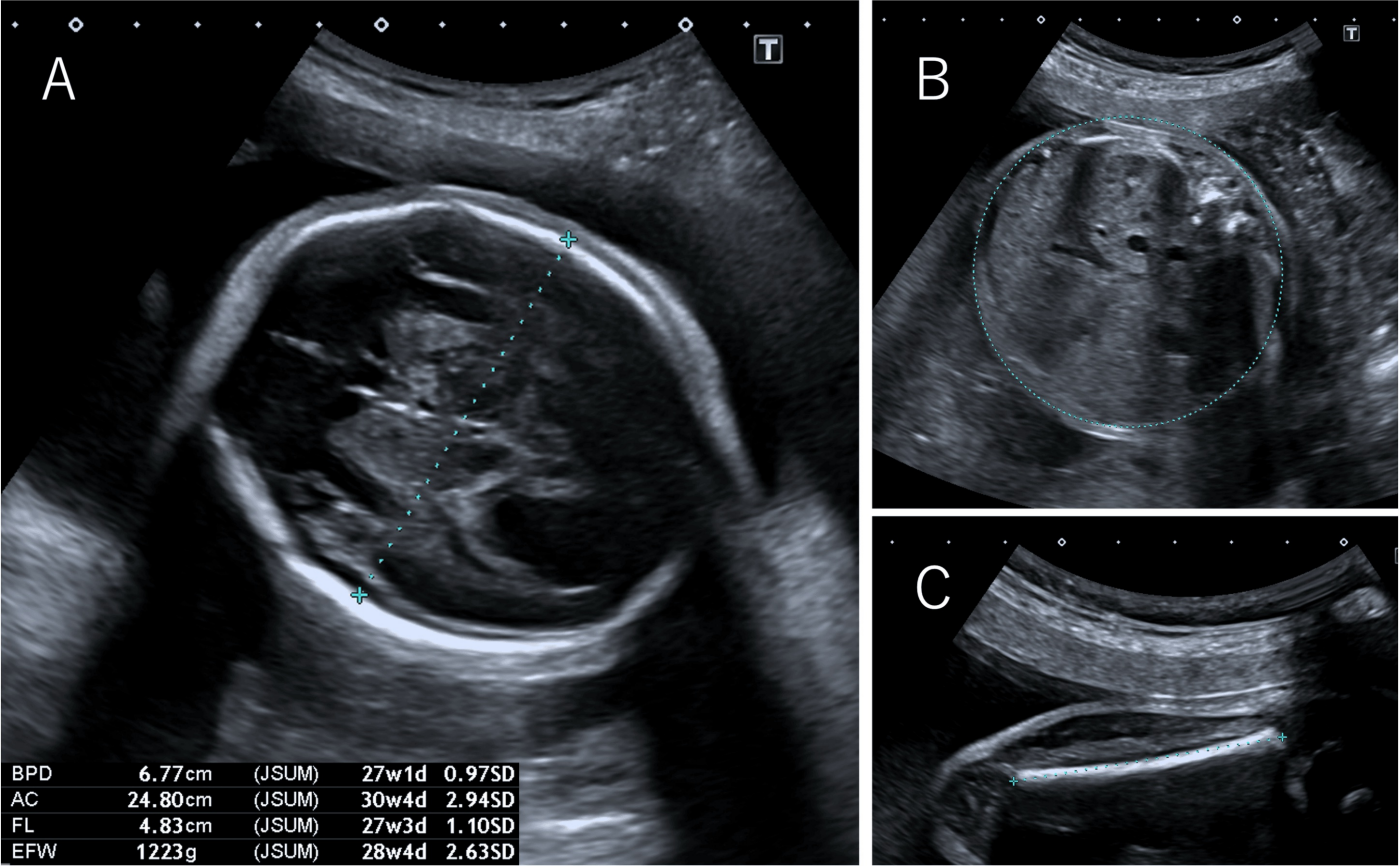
Ultrasound findings of the fetus at 24 weeks and four days of gestation. A. Biparietal diameter (BPD). B. Abdominal circumference (AC). C. Femur length (FL). The estimated fetal weight was 1.223 g (+2.63 standard deviation)
